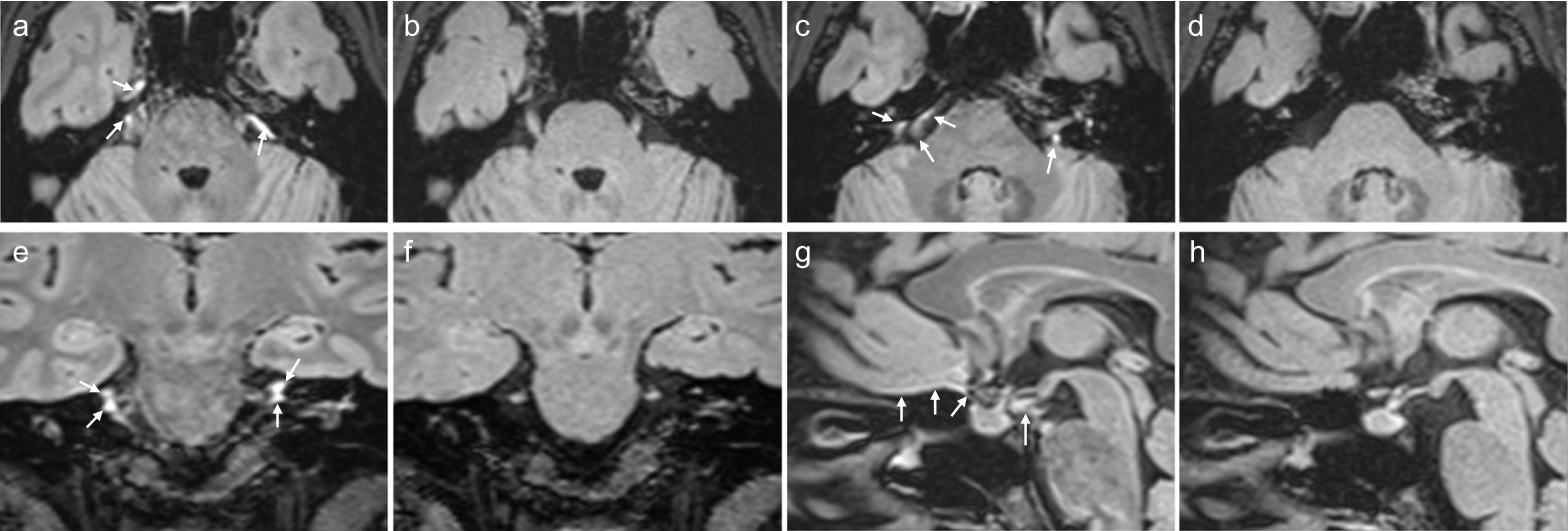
Comparison of 3D-FLAIR imaging with a T2-selective inversion pulse to that obtained with a conventional inversion pulse in a healthy female volunteer in her 40 s. MR imaging was performed at Siemens 3 Tesla using a 32-channel array head coil similar to that used in the article by Albayram et al.^3^. The scan parameters are also comparable to those used in the article by Albayram et al. In the 3D-FLAIR imaging with a T2-selective inversion pulse (a, c, e, g), similar contrast between gray/white matter is achieved as in the images shown in the article by Albayram et al. even with the same repetition time of 5000 msec. In 3D-FLAIR imaging with a conventional inversion pulse (b, d, f, h), less contrast between gray/white matter is provided than observed in the images of the article by Albayram et al. using the same repetition time of 5000 msec. High signal intensity, which corresponds to the areas presumed to be lymphatic tissues in Fig. 4 of the article by Albayram et al. (arrows in a, c, e, g) is only visible in the 3D-FLAIR images obtained with a T2-selective inversion pulse.
